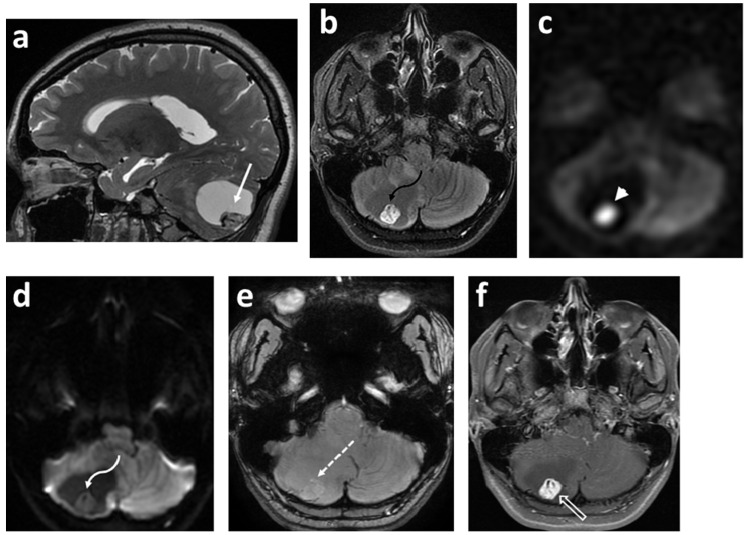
Sixteen-year-old female presented with headaches awakening her from sleep. Sagittal T2 (a); axial FLAIR (b); axial ASL-PWI (c); axial DWI (d); axial SWAN (e); and axial T1 fat saturated post contrast (f) images. There is a large cystic lesion within the right cerebellar hemisphere (solid white arrow) with a solid component within its posterior and inferior aspect (curved black arrow). The solid component of the tumor is hyperintense on T2/FLAIR images with multiple prominent flow voids indicating a highly vascular tumor. There is marked increased perfusion (arrowhead) of the solid component without associated restricted diffusion (curved white arrow). There is no calcification or hemorrhage within the solid component (dashed arrow), with the solid component demonstrating intense enhancement (open arrow) on the post-contrast image. Features are highly specific for hemangioblastoma (pathology proven). Author’s institutional human ethics committee/institutional review board guidelines were followed for anonymized images.
